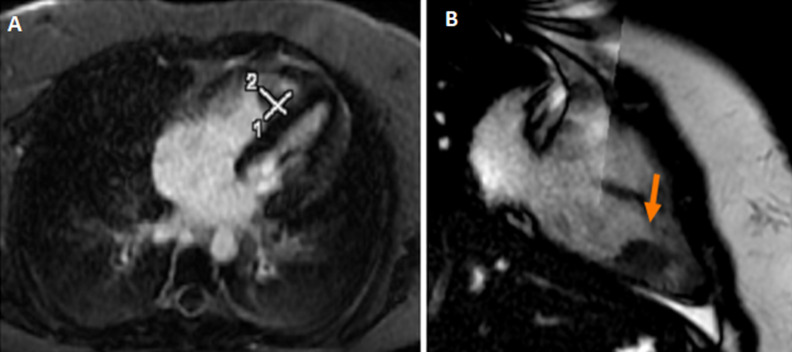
cardiac magnetic resonance imaging; thrombus in the lateral wall of the right ventricle (red arrow)
